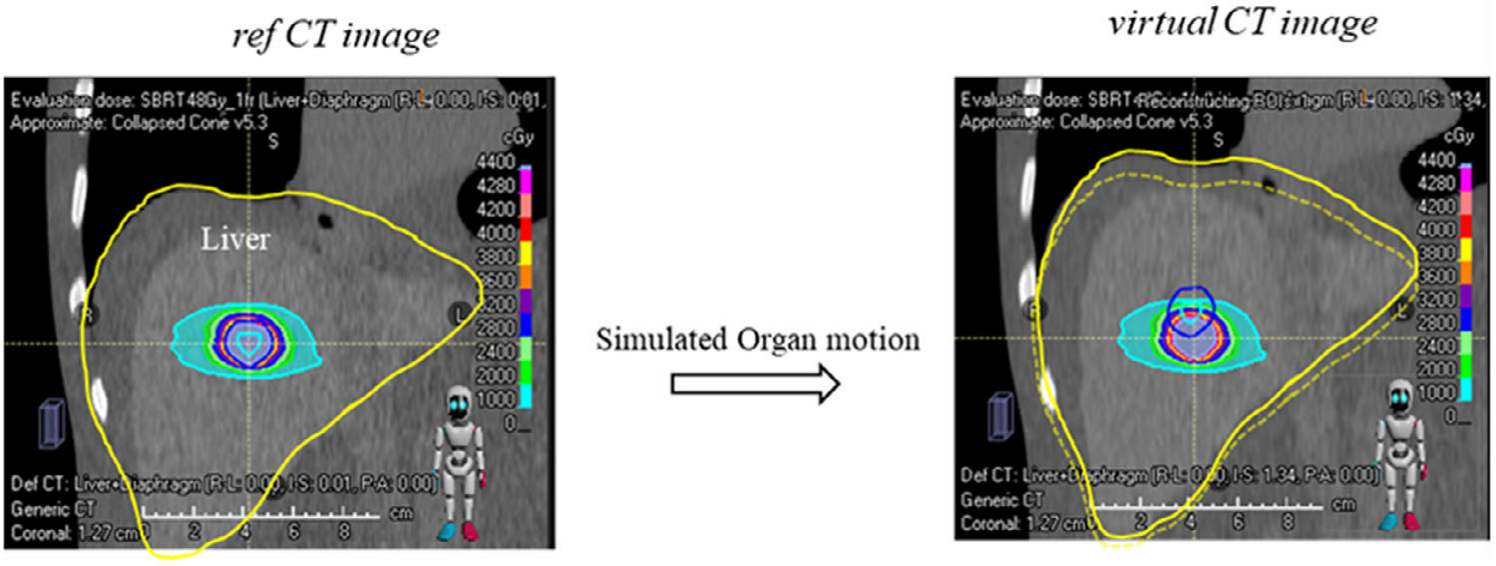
Synthesis of the virtual CT images from the reference CT images.
